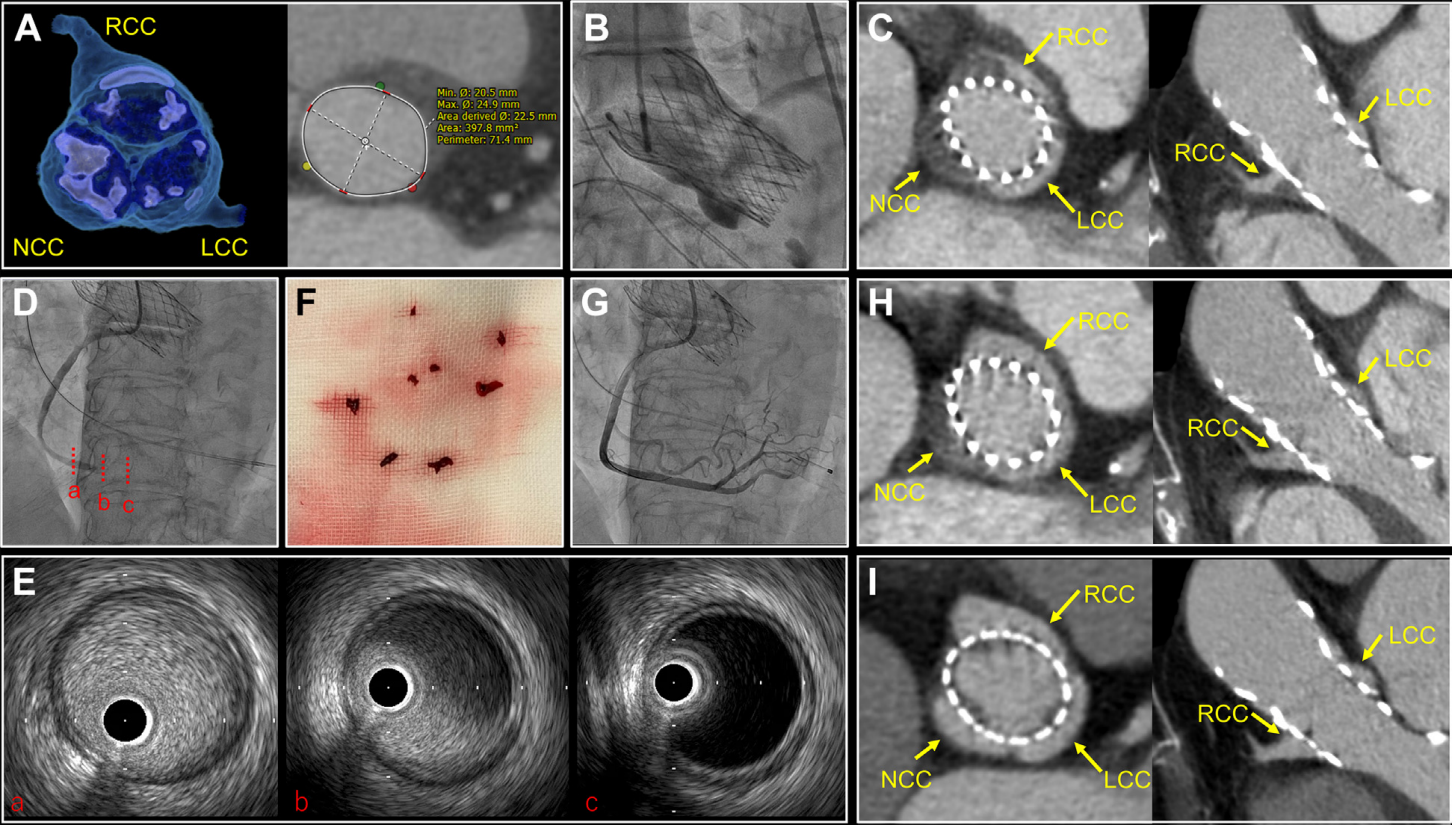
Coronary Embolization of Valsalva Thrombus Following TAVI (A) Preprocedural computed tomography (CT) images of aortic valve. (B) Aortography after 29-mm Evolut FX implantation. (C) CT images obtained the day after transcatheter aortic valve implantation (TAVI showing a thrombosis of the right coronary cusp and noncoronary cusp). (D) Emergent coronary angiography and (E) intravascular ultrasound showing thromboembolism of the right coronary artery. (F) Picture of aspirated thrombi. (G) Final coronary angiography showing successful revascularization. (H) CT images obtained immediately after percutaneous coronary intervention showing disappearance of right coronary cusp thrombus. (I) CT images 1 month following oral anticoagulation showing disappearance of noncoronary cusp thrombus. LCC = left coronary cusp; NCC = noncoronary cusp; RCC = right coronary cusp.
